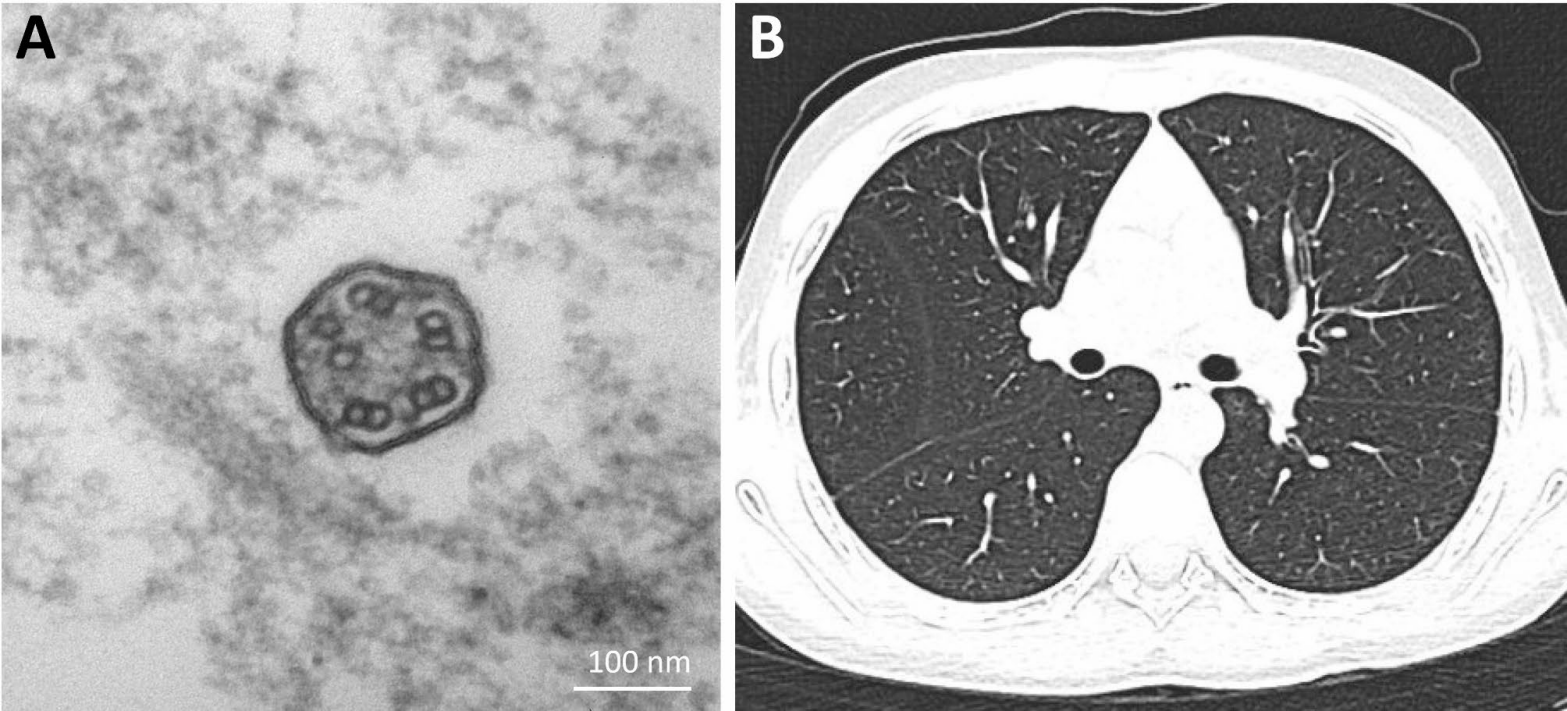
A: The TEM results of a PCD patient (No. 37 in e-Table 1) showed central pair absent and microtubular disorganization in bronchial cilia; B: Chest CT scan of a CF patient (No.7 in e-Table 1) indicates slight bronchiectasis in both lungs, especially in the upper lobes
